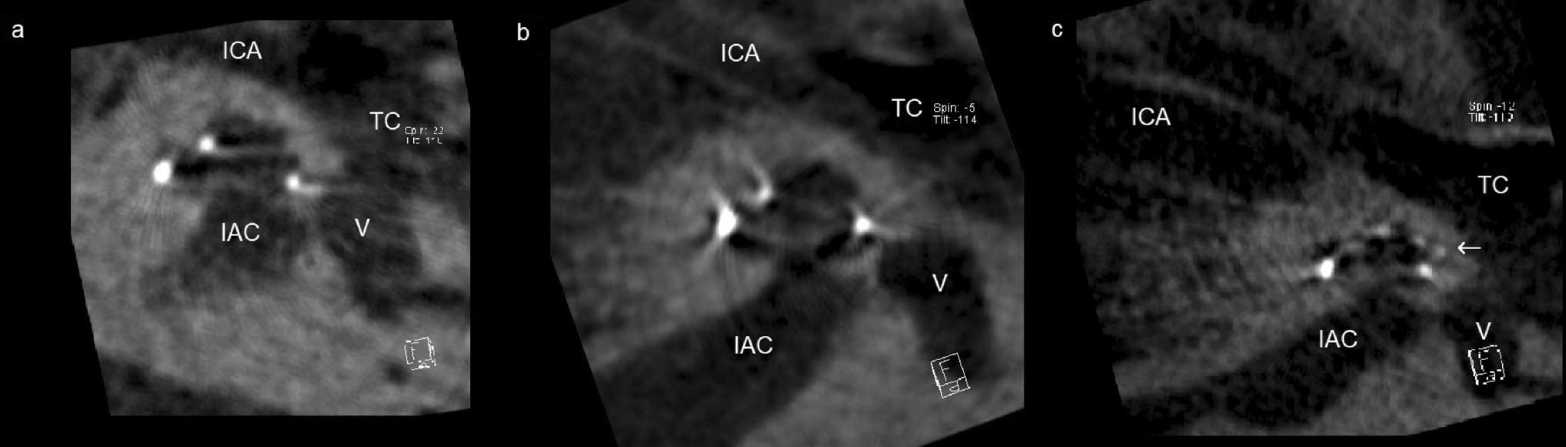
a CB-CT image of the Flex26 inserted in scala tympani without any signs of dislocation. b Flex28 inserted in scala vestibuli via cochleostomy. c FlexSoft inserted in scala tympani with a dislocation (arrow) to scala vestibuli. *ICA* internal carotid artery, *IAC* internal acoustic canal, *TC* tympanic cavity, *V *vestibulum)
